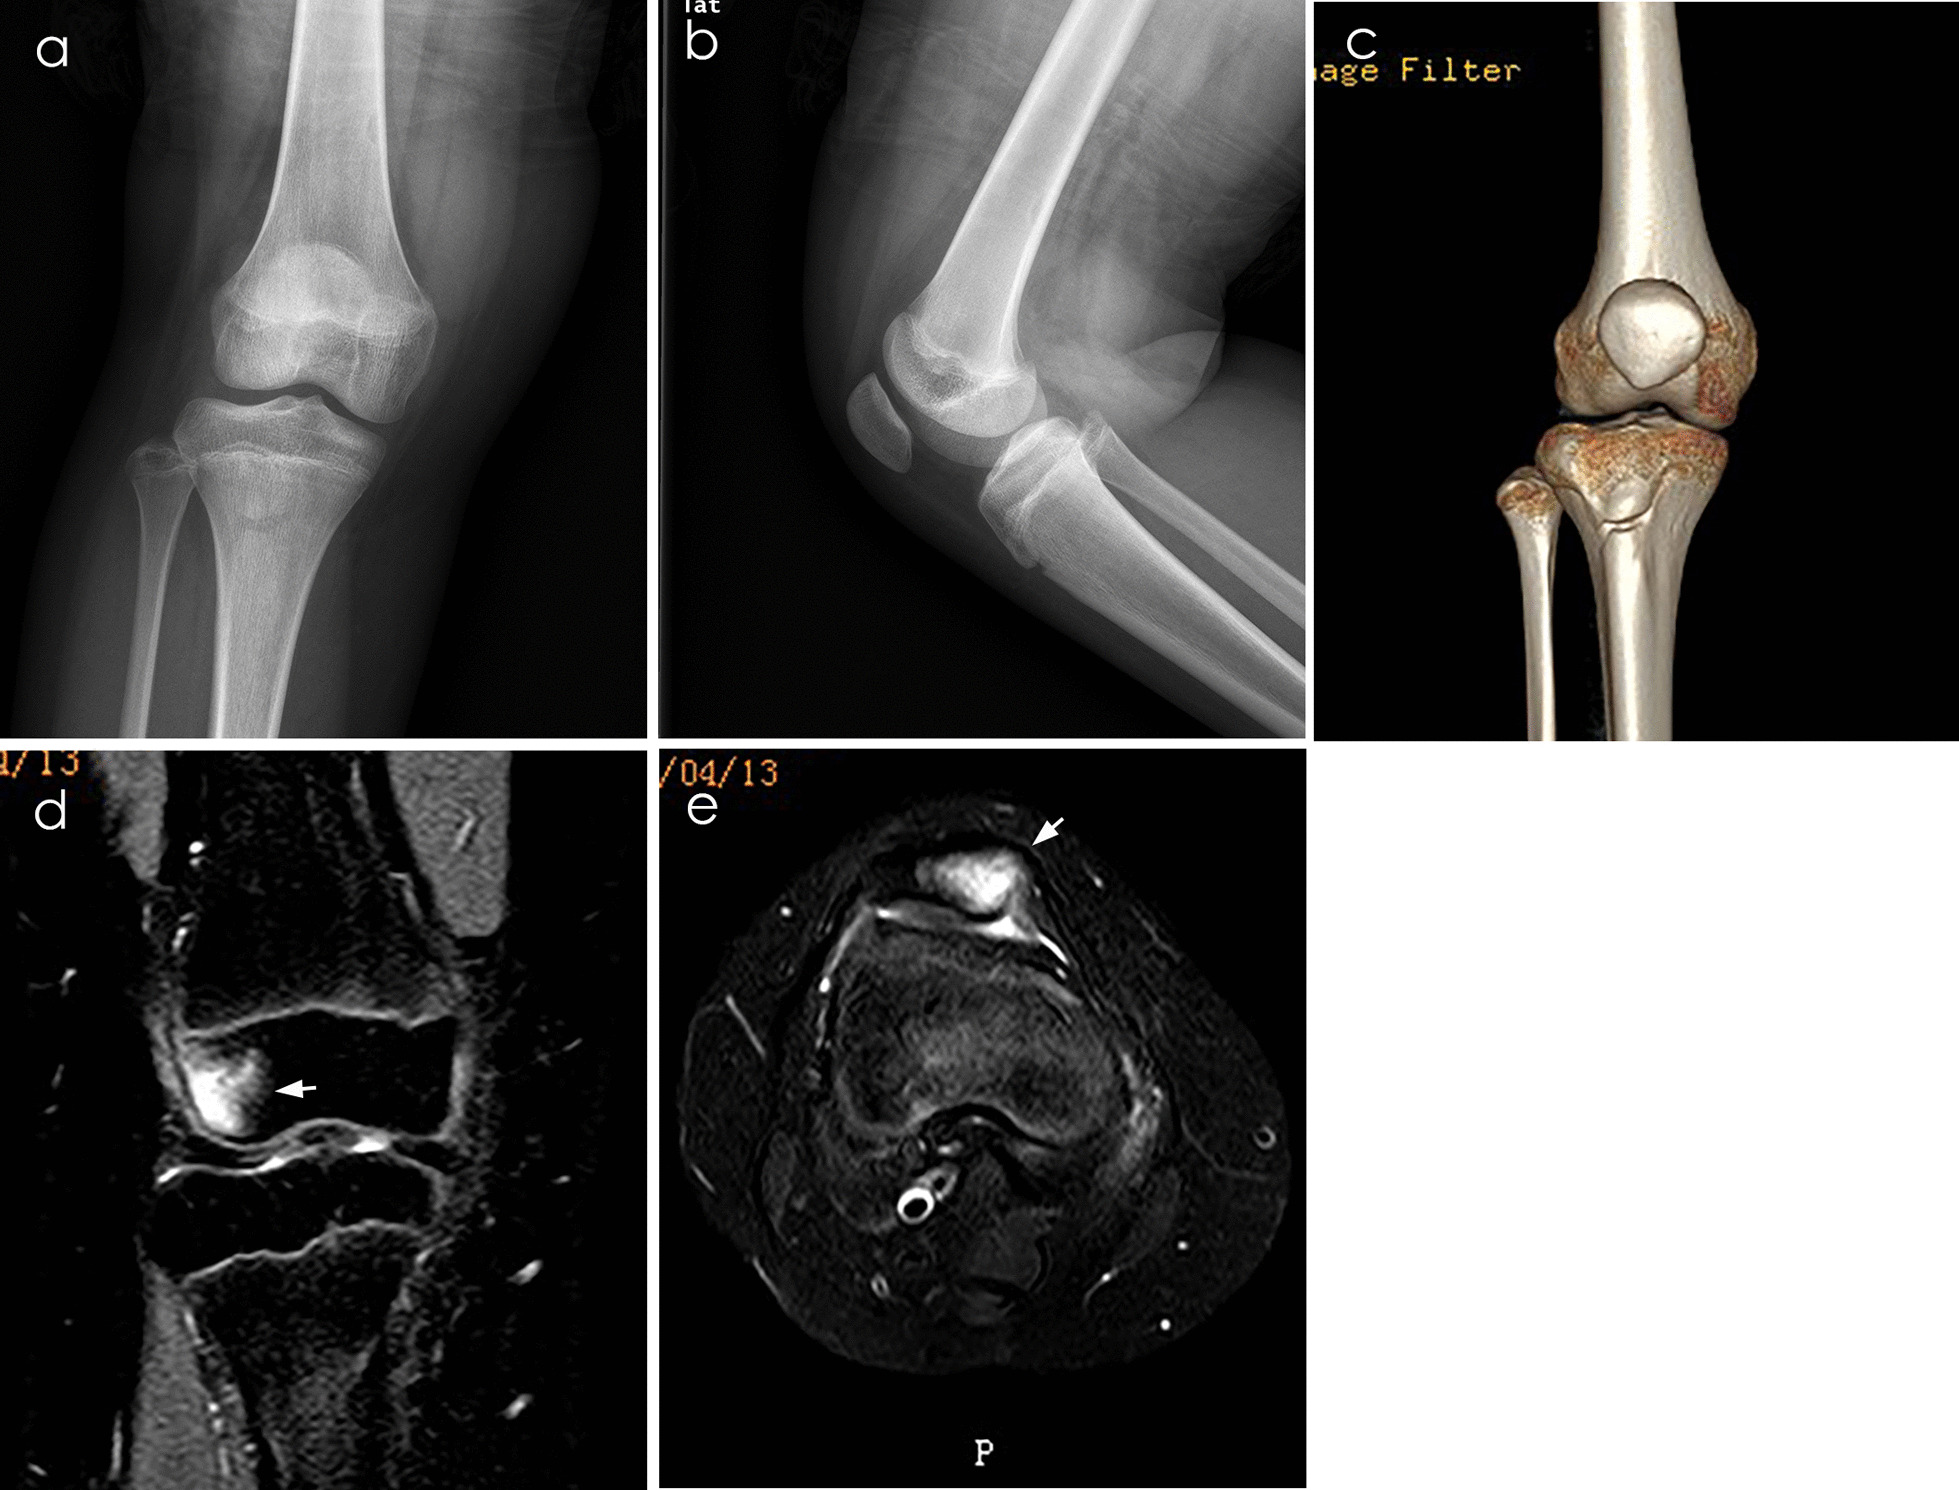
Plain radiograph, CT, and MRI of a 10-year-old girl with right traumatic patellar dislocation. a, b Anteroposterior and lateral knee radiograph did not show the patella fracture line. C CT after reconstruction did not show the patella fracture line. d, e Coronal and cross view of T2-weighted MRI showed bone contusion on the medial side of the patella of the lateral femoral condyle
